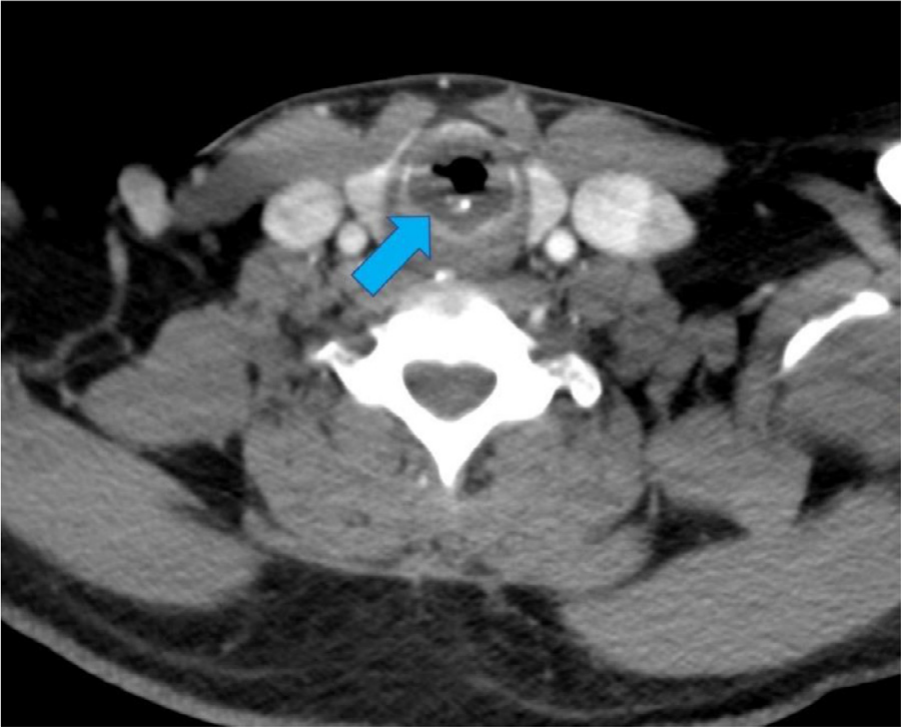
Axial contrast-enhanced CT of the neck with prominent circumferential thickening (blue arrow) of the subglottic airway with calcifications and associated airway narrowing.
